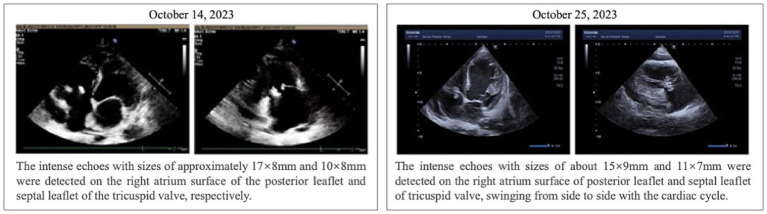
Results of echocardiography in patients.
